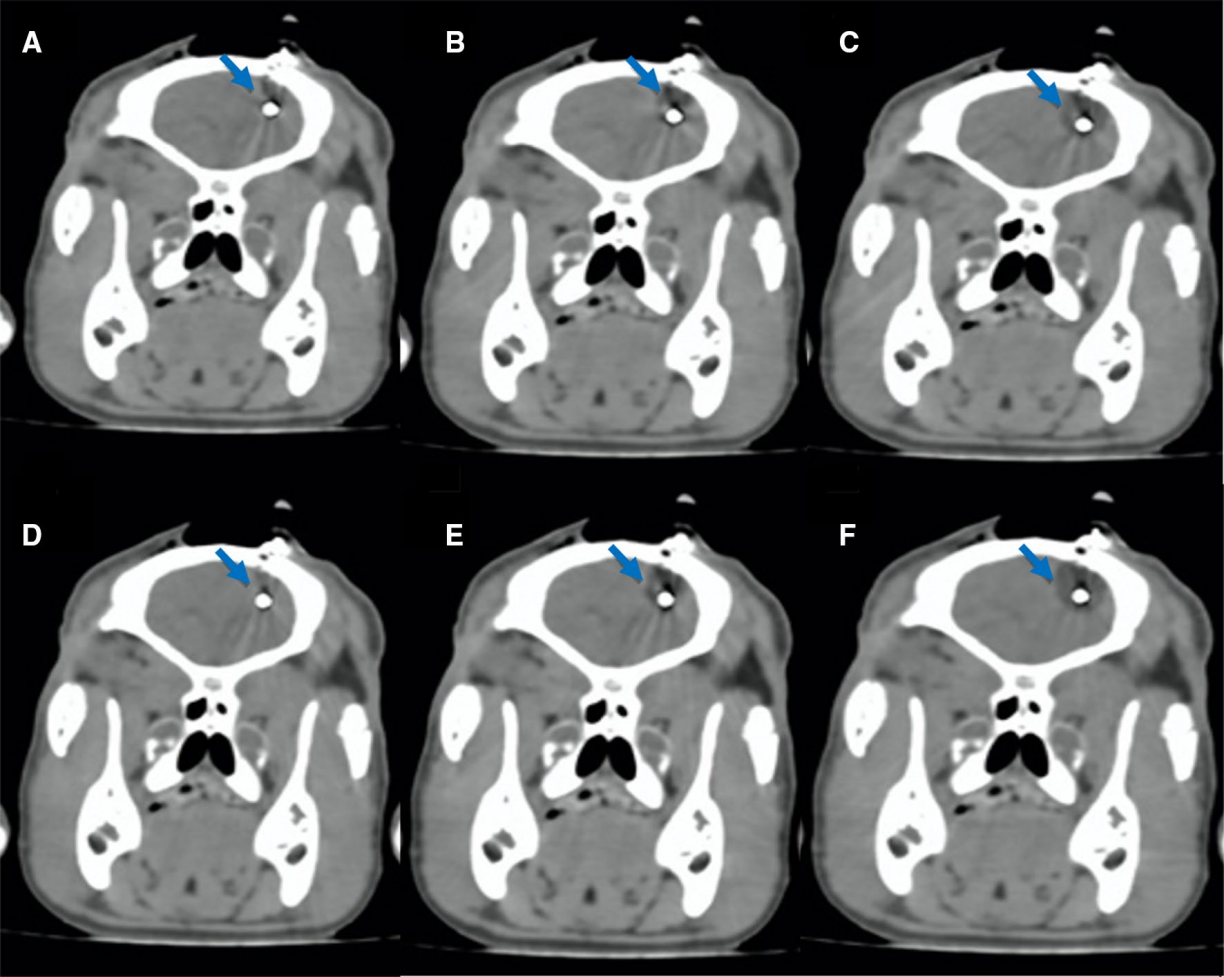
Metal artefact reduced coronal section CT scans of the brain after cryoablation (blue arrow). (A–C) Ice formation during the first freeze cycle; (A) after 0.5 min, (B) after 1.5 min, and (C) after 3 min. (D–F) Ice formation during the second freeze cycle; (D) Lesion size after 0.5 min, (E) after 1.5 min, and (F) after 3 min. No artefact caused by the probe was seen after metal artefact reduction.
